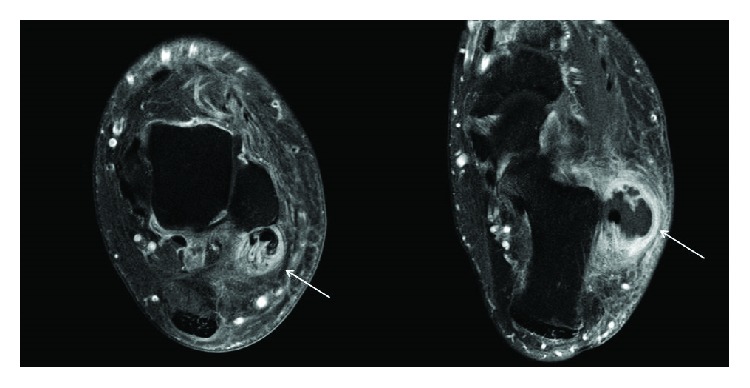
T2-weighted axial magnetic resonance images demonstrating a soft tissue tumor along and around the peroneal tendons with hypo- and hyperintense central areals and an inhomogenous, diffuse uptake of the contrast agent (arrows), highly suspicious for a synovial sarcoma.
